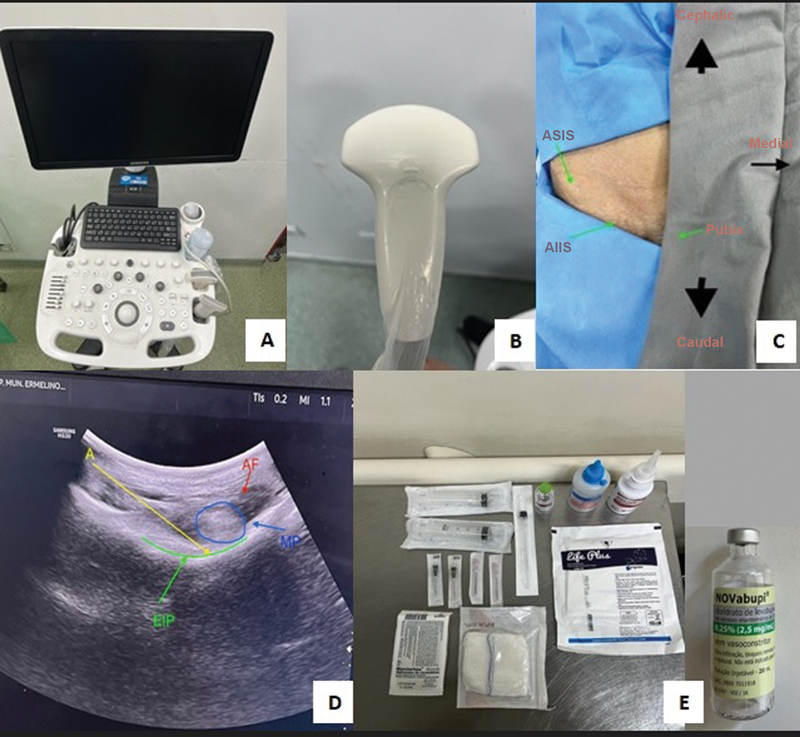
Ultrasound and patient preparation for the PENG block. (
